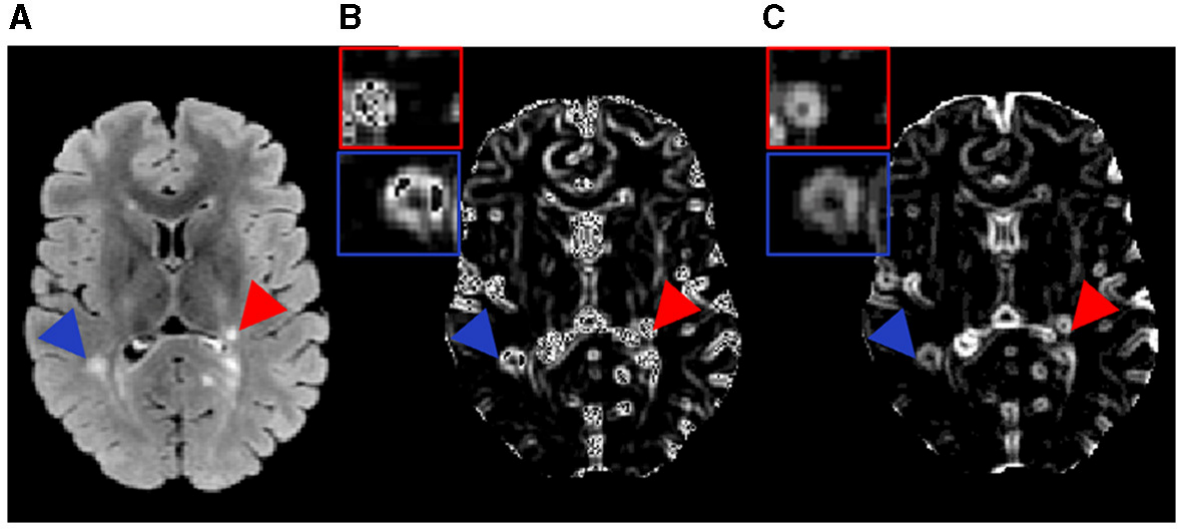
Example MR images and the texture feature maps from another RRMS participant examined in this study. Shown are a FLAIR image (A) as well as the resulting texture contrast (B) and texture dissimilarity (C) maps with corresponding lesions identified as severely demyelinated (red) or highly remyelinated (blue). Brighter signal indicates greater texture value and more heterogeneity in the maps. The participant has a disability score of 1.5 and disease duration of 9.5 years.
